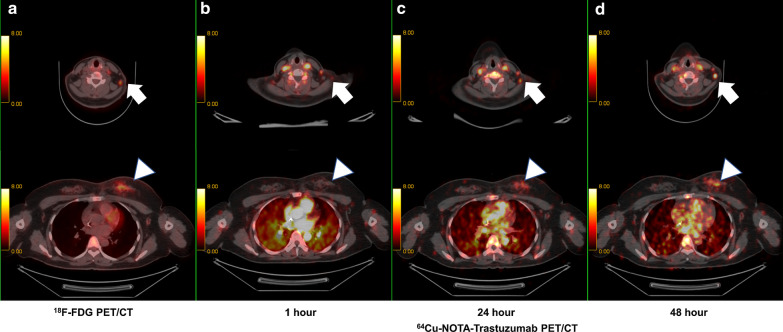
^64^Cu-NOTA-Trastuzumab PET images of HER2-positive breast cancer (arrowheads, lower row) and metastatic lymph node (arrows, upper row). The primary tumor and metastatic lymph nodes were clearly observed by ^18^F-FDG PET/CT (a) and ^64^Cu-NOTA-Trastuzumab PET/CT (b–d). In HER2-expressing lesions, the uptakes of NOTA increased over time to 48 h after injection
